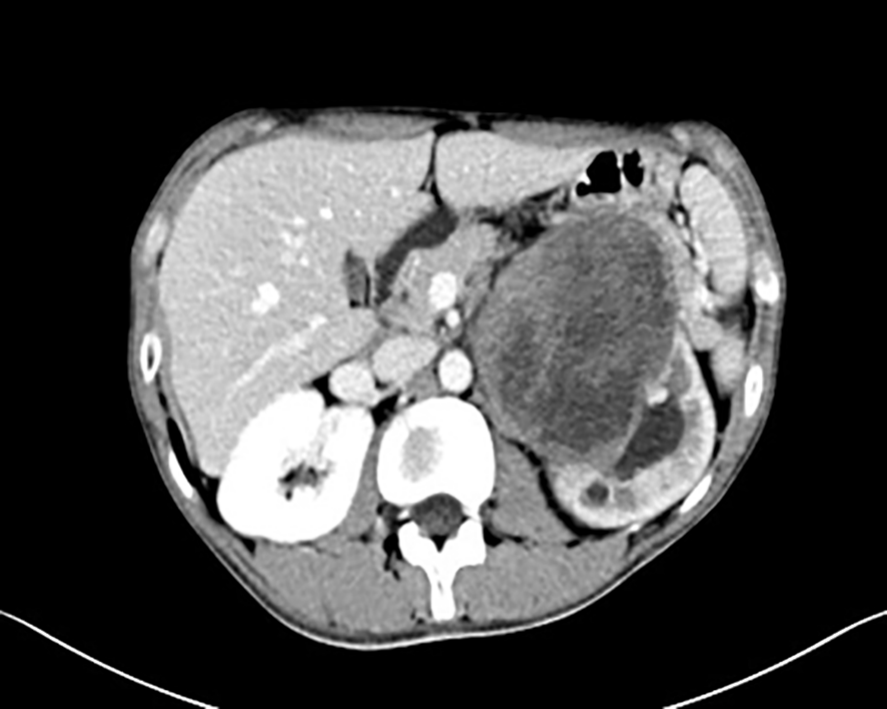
CT imaging characteristics. Intra-abdominal mass in the left upper quadrant.
